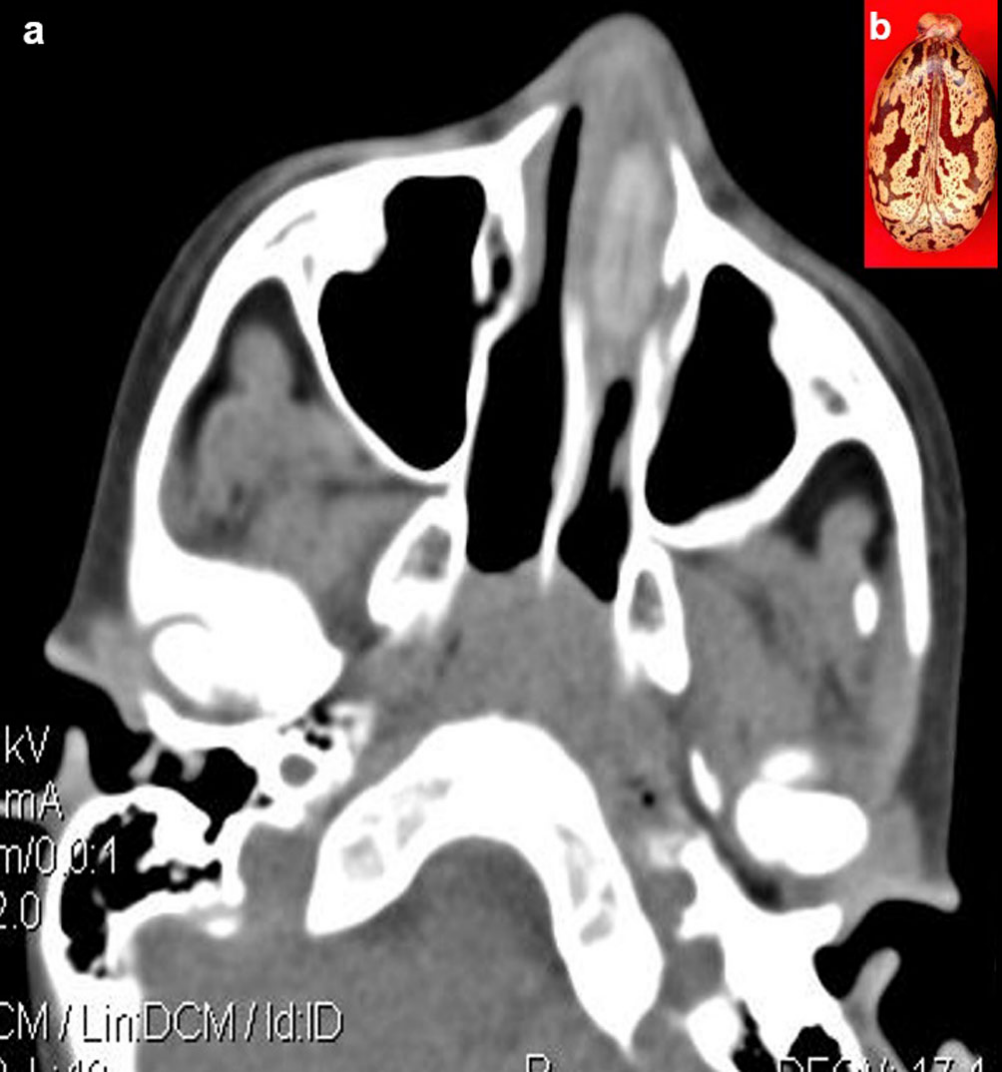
a & b: CT PNS axial section revealed a non-enhancing hyperdense foreign body in the left nasal cavity (a) Castor seed (b).
